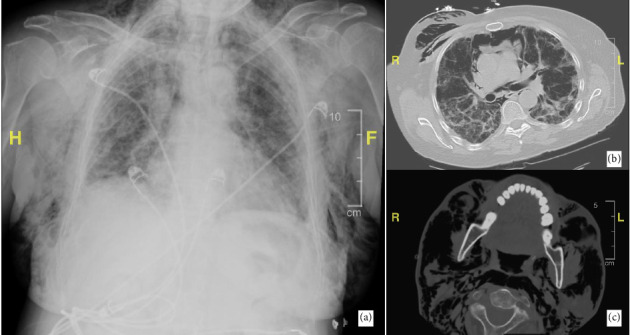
(a) Diffuse subcutaneous emphysema with bilateral infiltrates on chest x-ray. (b) CT chest showing subcutaneous emphysema and pneumomediastinum with associated lung infiltrates and areas of scarring bilaterally. (c) CT neck showing subcutaneous emphysema involving the neck with extension into the head.
